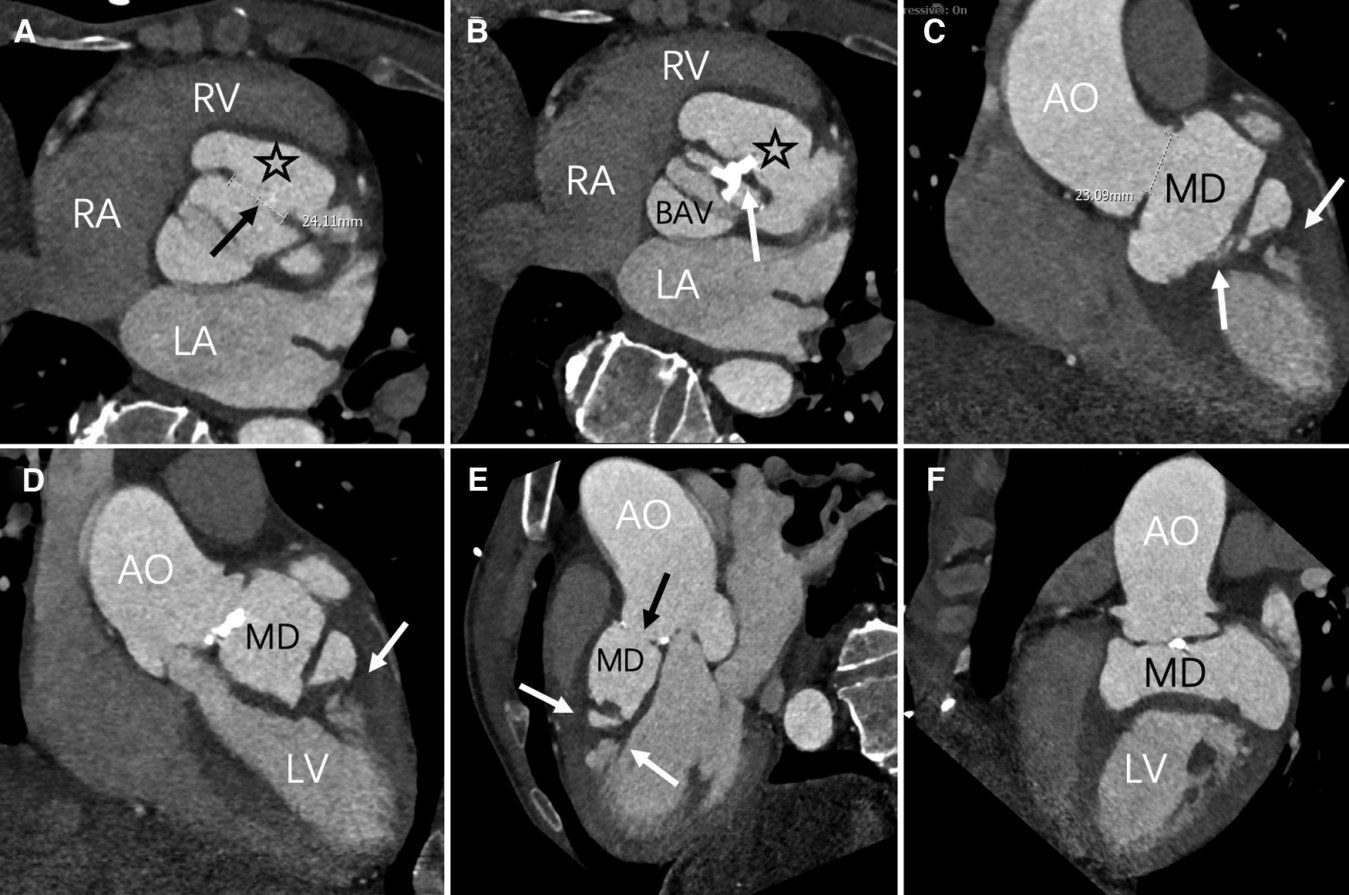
Axial position, contrast-enhanced CT imaging reveals the maximum aperture of the sinus of Valsalva aneurysm to be 2.4 cm (black arrow). The myocardial dissection originates from the fused left and right coronary sinuses (asterisk). A bicuspid aortic valve anomaly is evident, with valve leaflet thickening and calcification (white arrow) (C–F) multiPlanar Reconstruction (MPR) exhibits irregular MD, fusion of the left and right coronary sinuses (black arrow), and high-density opacities within the ventricular wall and interventricular septum (white arrow). LA, left atrium; LV, left ventricle; RA, right atrium; RV, right ventricle; AO, Aortic; MD, Myocardial dissection; BAV, bicuspid aortic valve.
